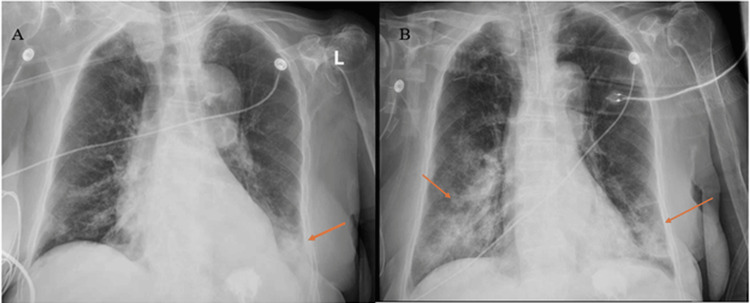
Initial X-rays of the chest CXR on admission showing left lower lobe infiltrate with small left pleural effusion (left) and CXR performed 24 hours after admission, with worsening bilateral pulmonary infiltrates (right).
